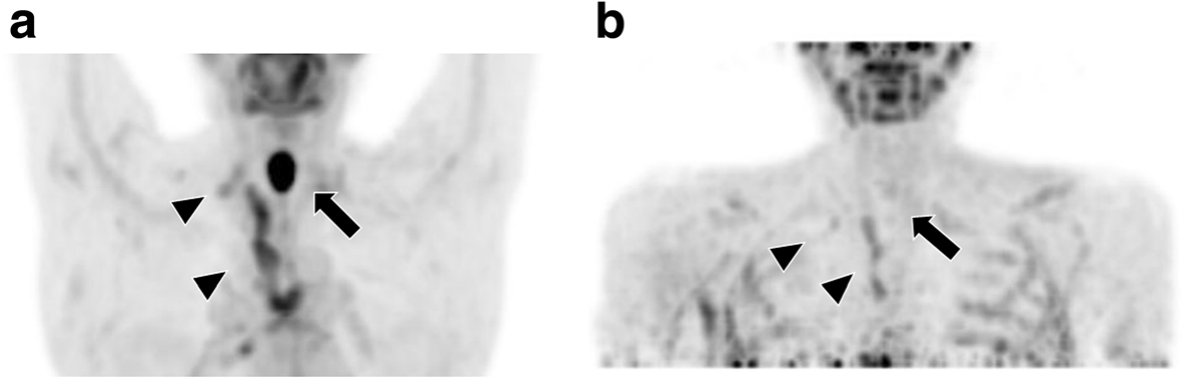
FDG-PET/CT images obtained before (a) and after (b) chemotherapy in case 2. a Images obtained before chemoradiotherapy show FDG accumulation in the primary cancer (arrow; SUV max: 23.1) and in the mediastinal lymph nodes (arrow head; SUV max: 5.45). b Images obtained after chemotherapy show a disappearance in FDG uptake in the primary cancer (arrow) and a slight reduction in FDG uptake in the mediastinal lymph nodes (arrow head; SUV max: 3.26)
